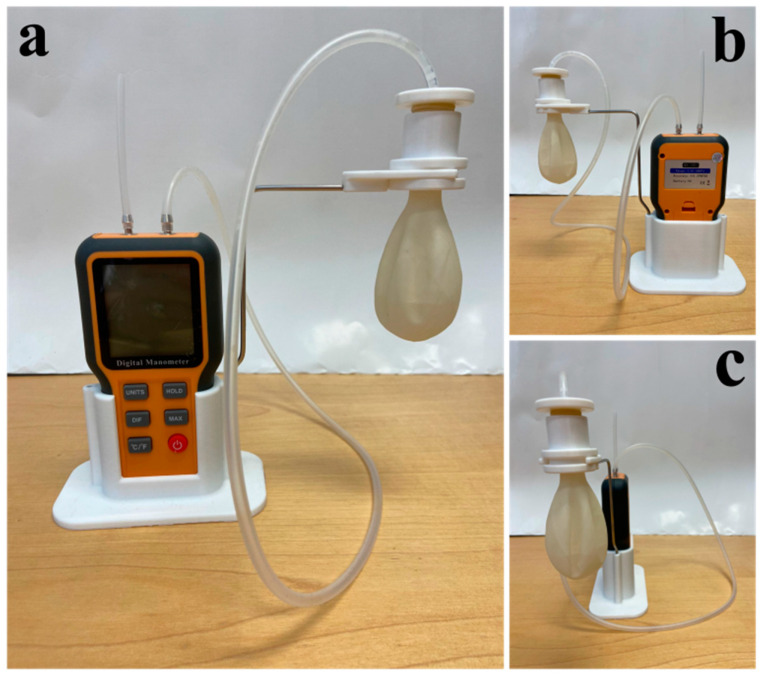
Picture of our new differential pressure measurement dynamometer with approximately 30 mL of water placed in the ultrasoft bulb; (a) front view; (b) back view; (c) side view. The digital differential manometer is the instrument with a yellow color sitting in a 3-D printed white polylactic acid (PLA) plastic stand. The chamber or “squeeze bulb” is hanging from a white plastic 3-D printed water trap retention chamber to protect any water that may overflow through the tube and into the digital instrument. The tube connected to the chamber is 5 mm internal diameter made of silicone plastic. To dangle the chamber, a 3 mm steel bar is used.
